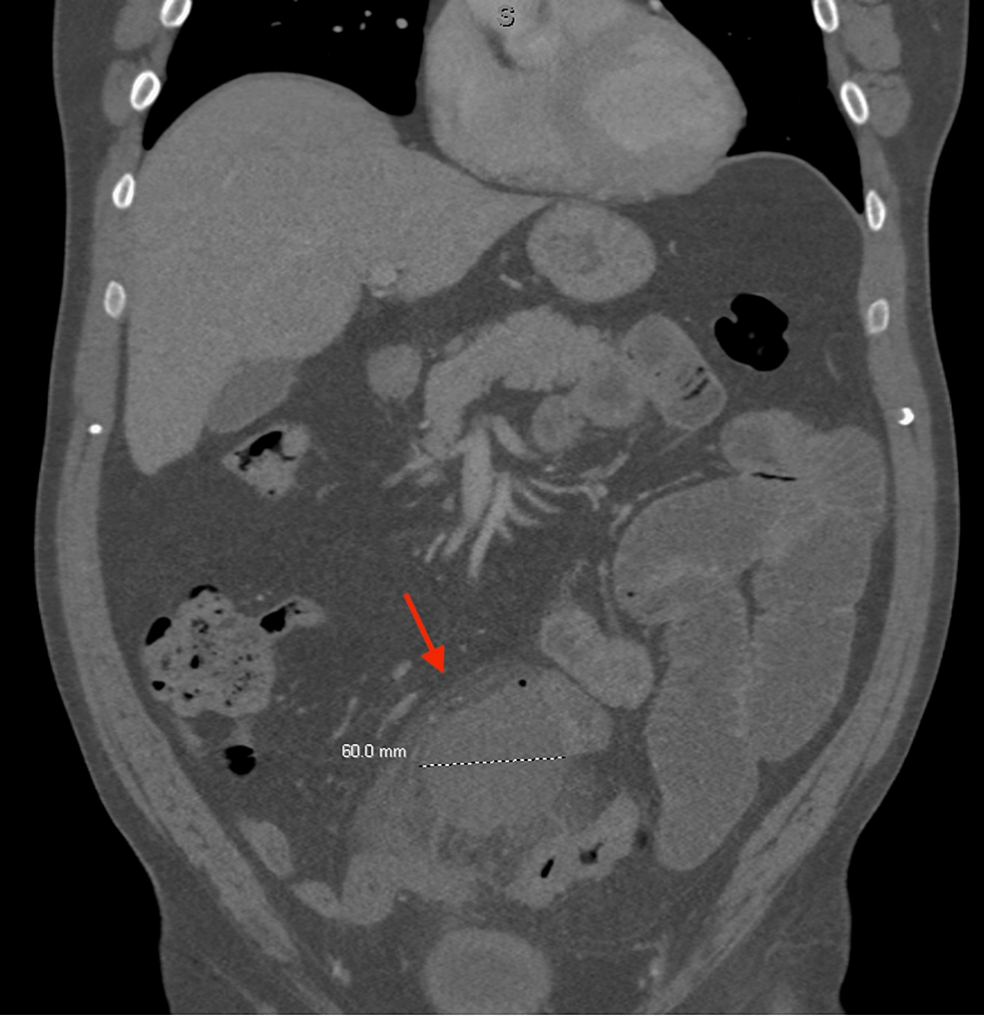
Coronal View of CT abdomen/pelvis with IV contrast CT: Computed tomography; IV: Intravenous The image depicts an abscess (50.8 x 60.0 x 60.0 mm, red arrow) with surrounding inflammation of the small bowel and sigmoid colon
